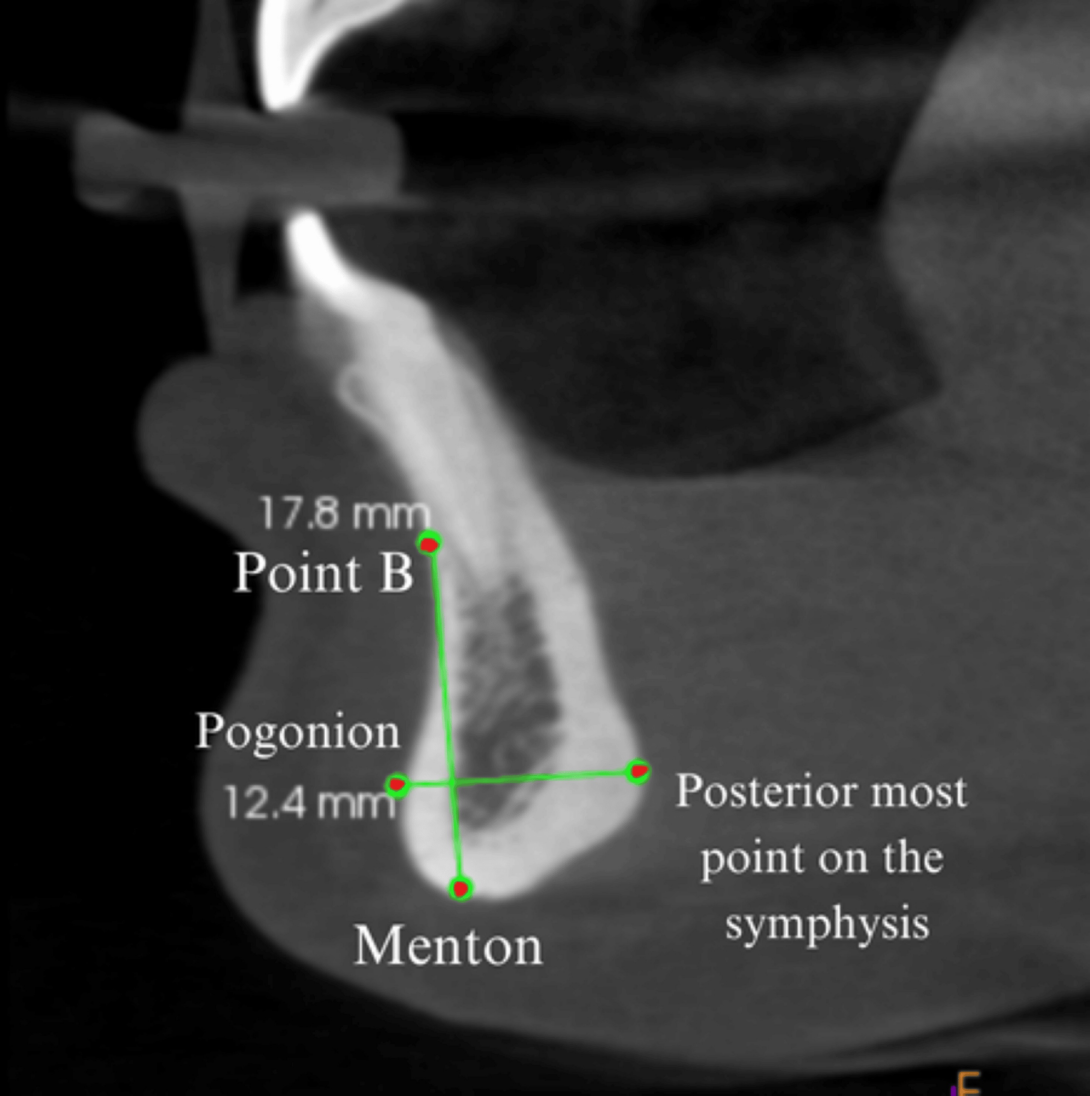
Mandibular symphysis measurements in mm. The symphyseal height was measured as the linear distance from point B to the menton. The maximum thickness was recorded as the horizontal distance between the pogonion and the most posterior point on the symphysis as assessed on the sagittal view of the CBCT scans. CBCT, cone-beam computed tomography This figure depicts cone-beam computed tomographic image of a patient included in this study and used with the patients' permission.
